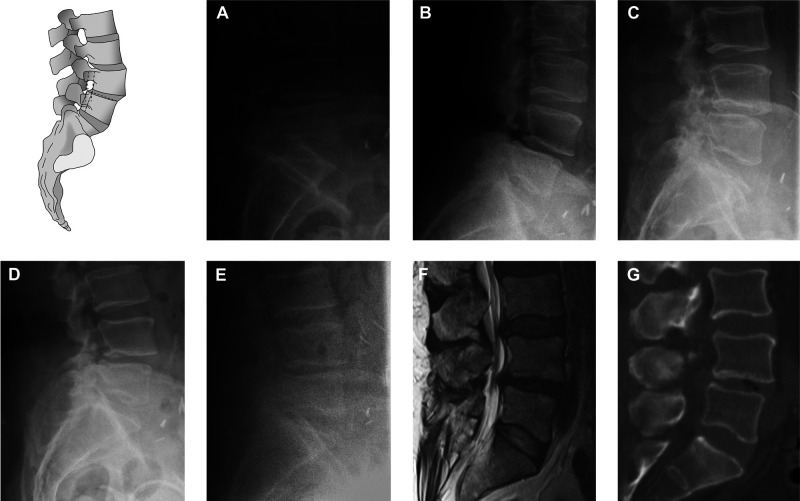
Comparisons of different imaging modalities. Sagittal translation was measured as indicated in the given pictogram. Slump siting (A), inclination (B), neutral (C), reclination (D), prone (E), MRI (F), and CT (G).
